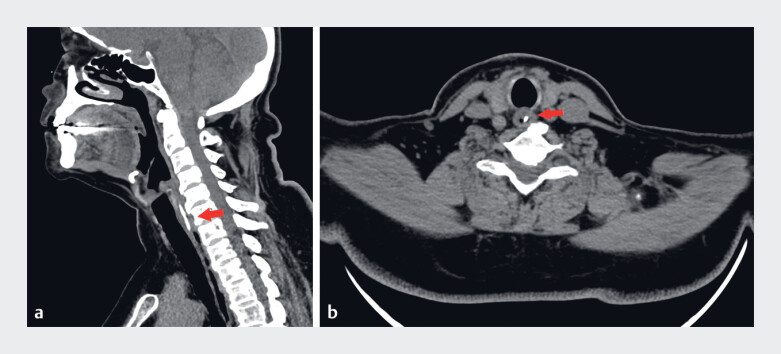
Preoperative computed tomography scan revealing the location of the fishbone (arrow).
